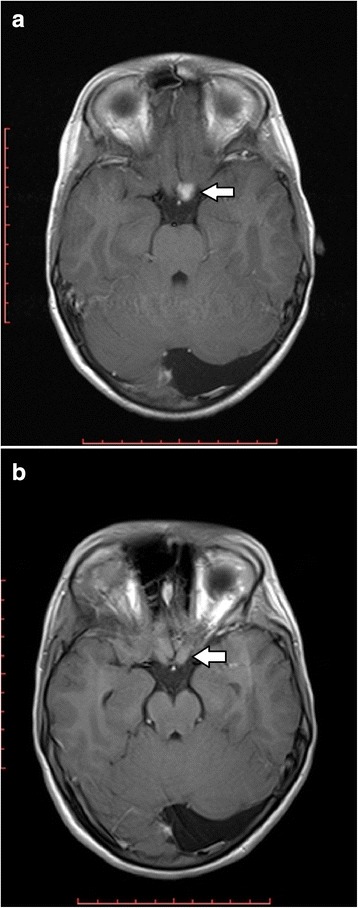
Spontaneous regression of an OPG in Patient 3. a A 516 mm^3^ left prechiasmatic optic nerve glioma on this patient’s first MRI at age 6.3 years. The tumour volume was unchanged over 2 MRI examinations during the next 2.2 years. b On re-examination at 9.3 years of age the OPG volume had decreased to 462 mm^3^, and the volume decreased again to 436 mm^3^ when the patient was 11.2 years old. The tumour volume was unchanged on the patient’s last MRI in this study at age 11.7 years
